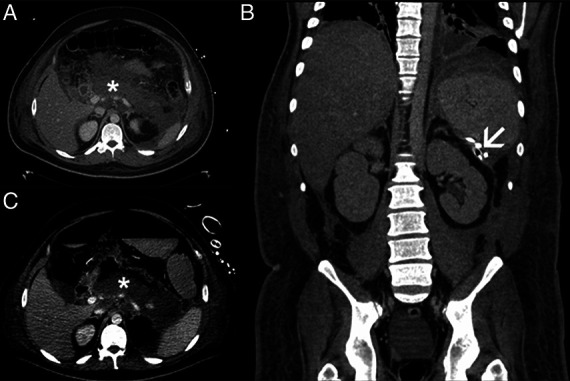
Extensive peripancreatic and retroperitoneal necrosis indicated by an asterisk (*) in the computed tomography of the patient (A, B). Retroperitoneal necrotic areas were treated with the installation of a transcutaneous drainage extending to the splenic hilum (as shown by the arrowhead) (C).
